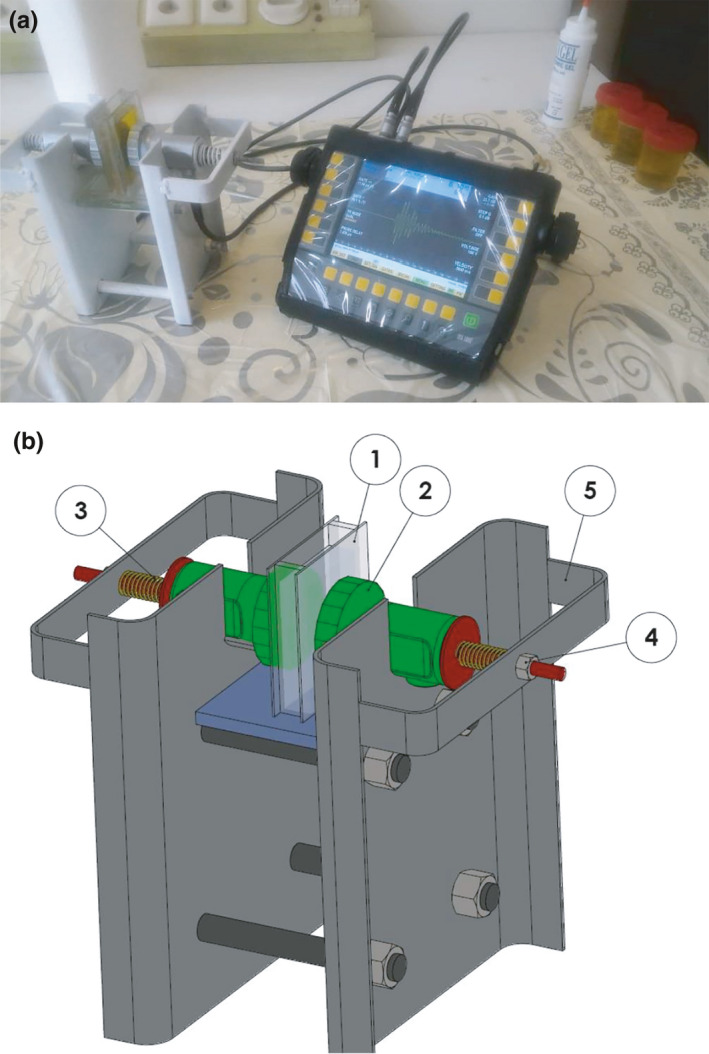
(a) Used diagnostic ultrasound device, (b) structure of probes holder (1. sample (oil), 2. ultrasonic probe (2MHz), 3. compression spring, 4. adjusting nut, and 5. main body)
